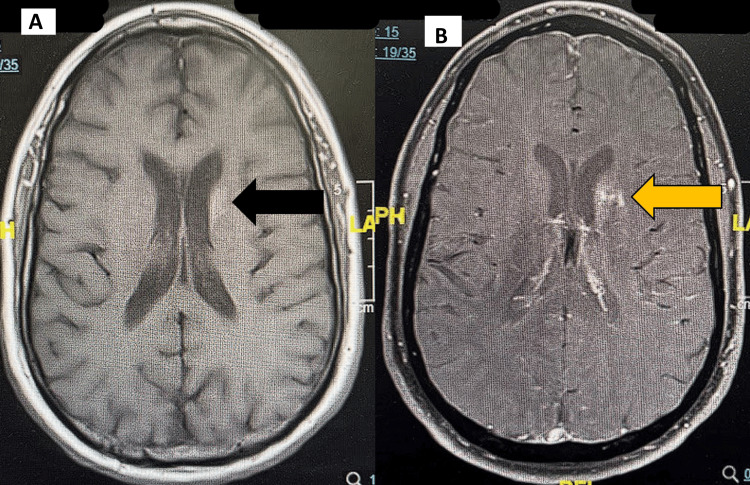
MRI T1 Sequence of the Brain (A): MRI T1 without contrast image of the brain. Black arrow pointing to hyperintensity in the left basal ganglia.
